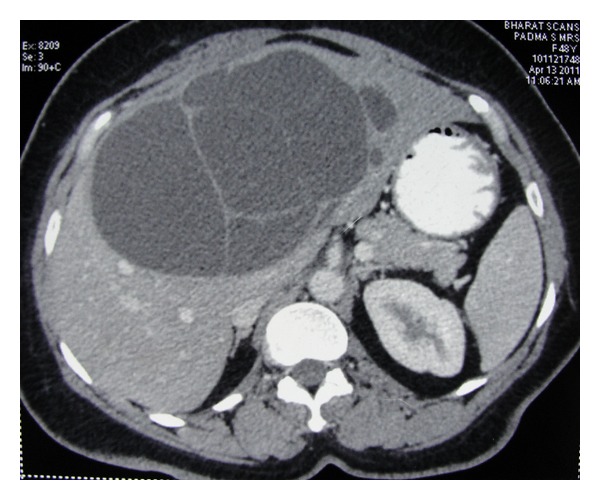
Contrast-enhanced CT scan showing large cystic lesion in liver with multiple-enhancing septae.
